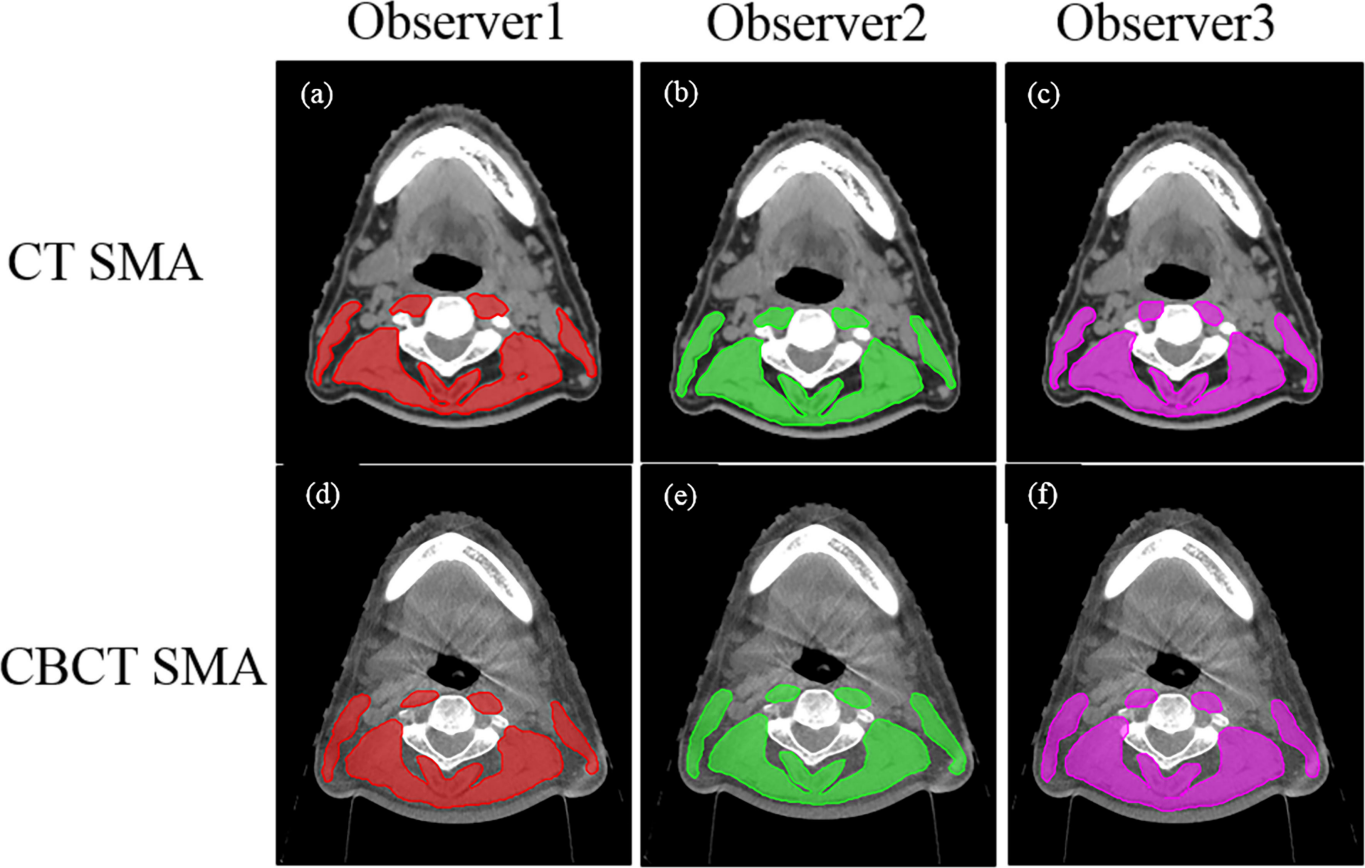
Computed tomography (CT) and cone-beam CT (CBCT) images identified by three independent senior radiation oncologists (observers 1, 2, 3). The upper panel (A–C) shows the CT skeletal muscle mass (29–150 HU) and the lower panel (D–F) shows the CBCT skeletal muscle mass (observer 1, red; observer 2, green; observer 3, pink).
